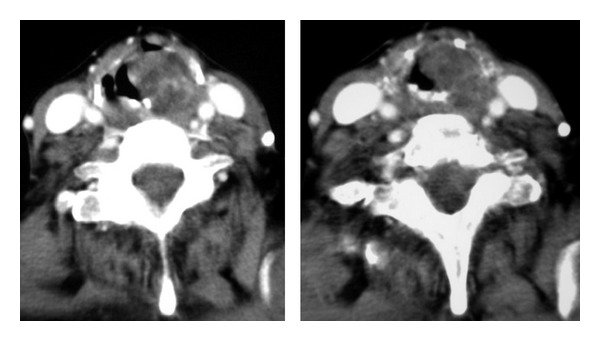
Axial CT scan showed an expansive mass occupying glottic space, originating from the left aryepiglottic fold.
